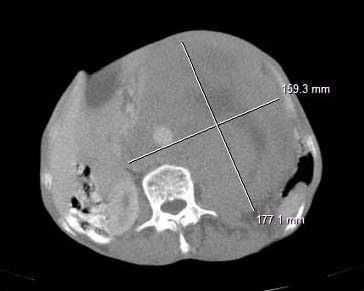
Computed tomography scan of the abdomen showed the retroperitoneal tumour.
